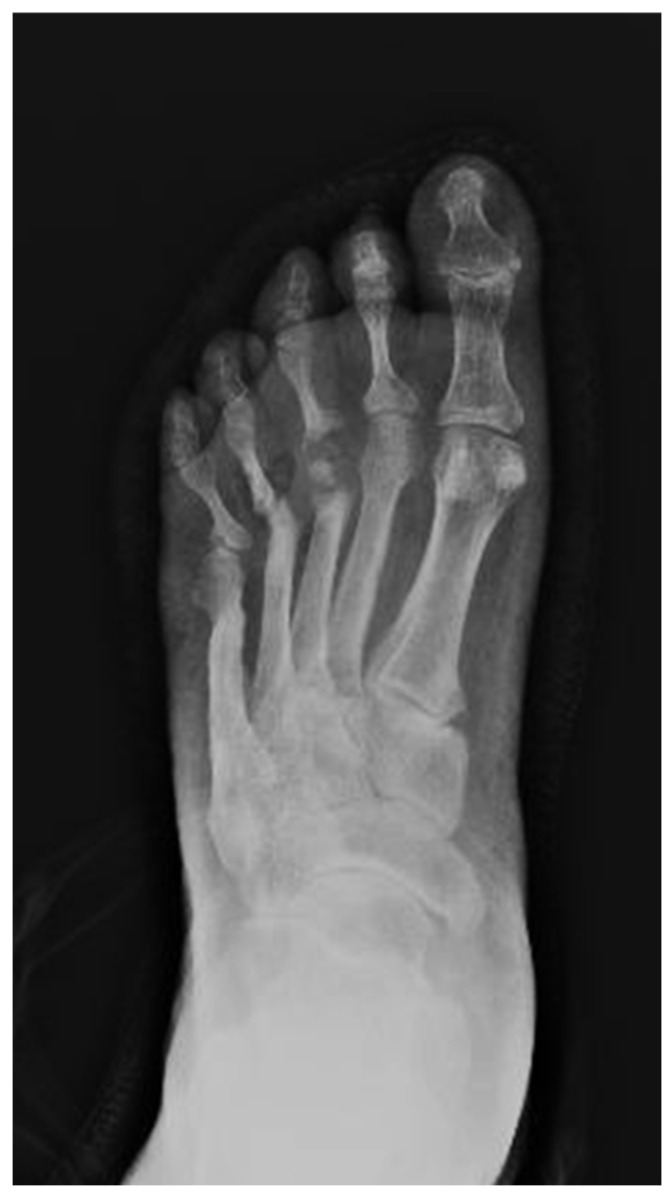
X-ray: Bony consolidation, fragmentation of the subchondral bone, fractures, dislocations, subluxations, osteopenia, and osteolysis.
